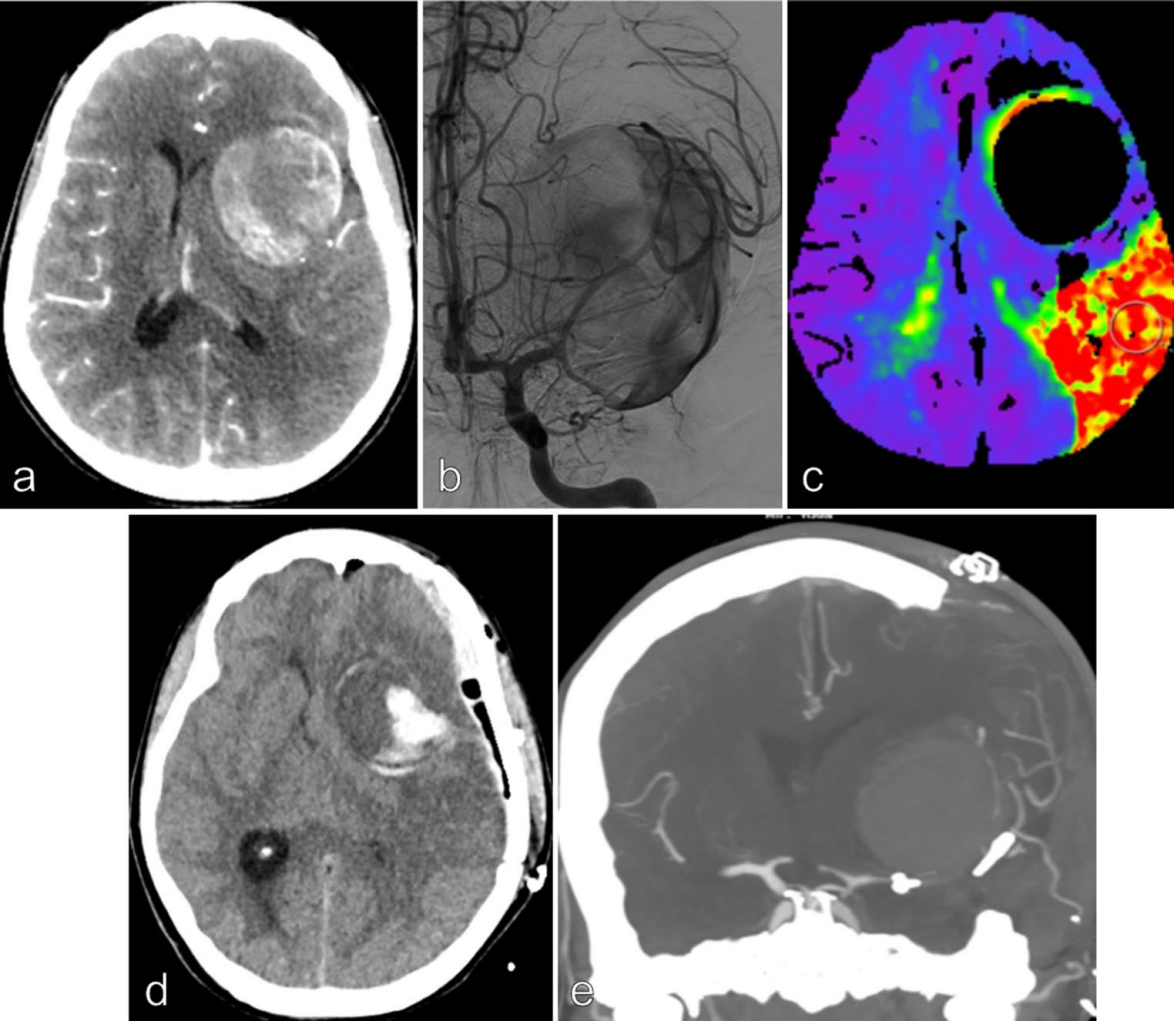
Patient 1, 41 yo female, smoker with a medical history of migraine, presented with headache and a 1-month history of moderate motor language disorder. CT scan, MRI/MRA and conventional angiography demonstrated a giant left MCA aneurysm injected only by the left temporal M2 segment. No significant edema, intrasaccular thrombus or wall calcifications were noted (a and b). The midline brain shift was 9 mm. A severe hypoperfusion in the left distal MCA territory was noted on the perfusion CT scan (c). A distal STA-MCA bypass was performed, followed by aneurysmal trapping and partial resection of the aneurysm sac. The patient was extubated after 24 h without any neurological deficit. 36 h after treatment, a rapid consciousness disorder with a left mydriasis occurred, related to a left hemispheric diffuse edema (d). A decompressive hemicraniectomy associated to a partial anterior temporal lobe resection was immediately done (e). A severe vasospasm appeared 5 days later and was partially improved by chemical angioplasty. The bypass was always patent. The patient improved, with a moderate language disorder but no motor deficit. The mRS score was 2 after 3 months
